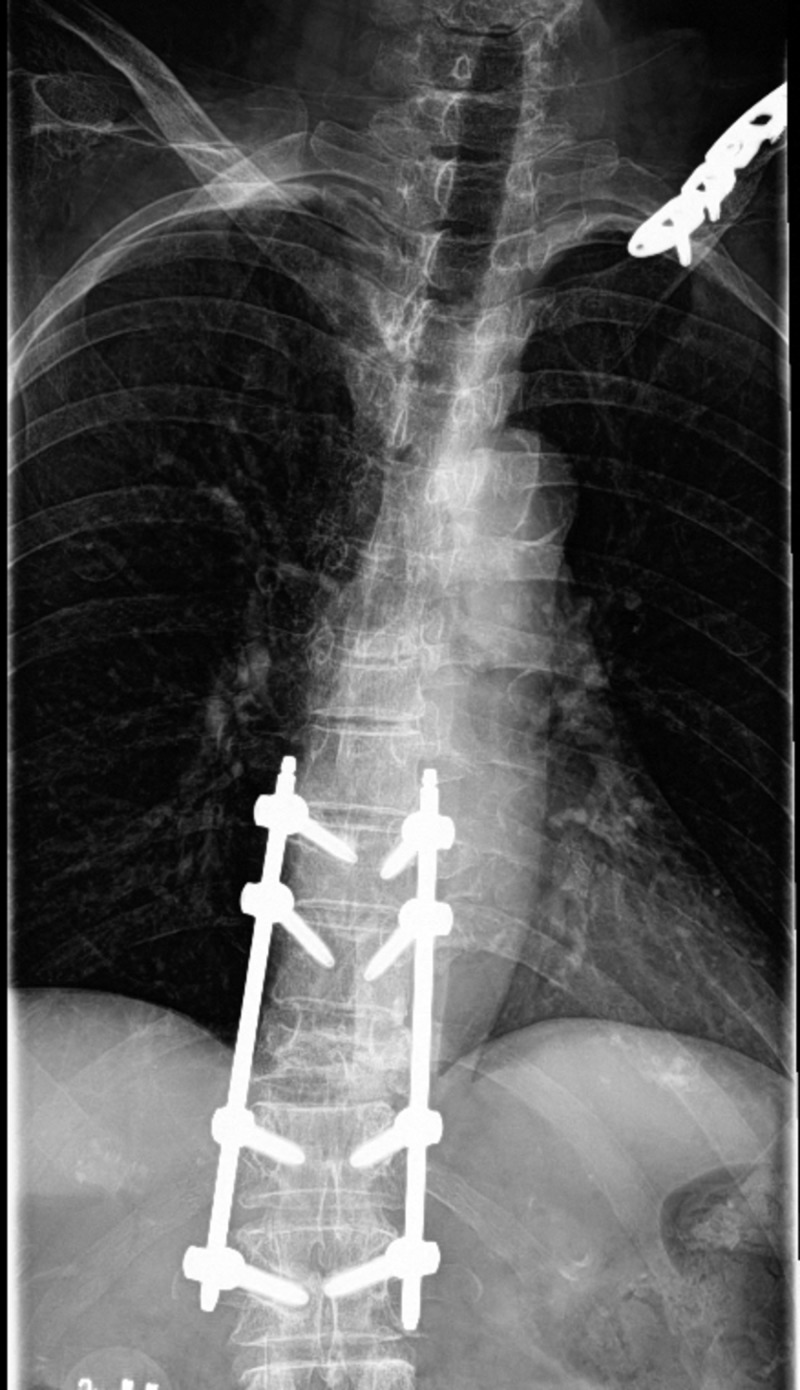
Six months post-operative thoracic spine X-ray with intact hardware without lucency, left clavicular plate
